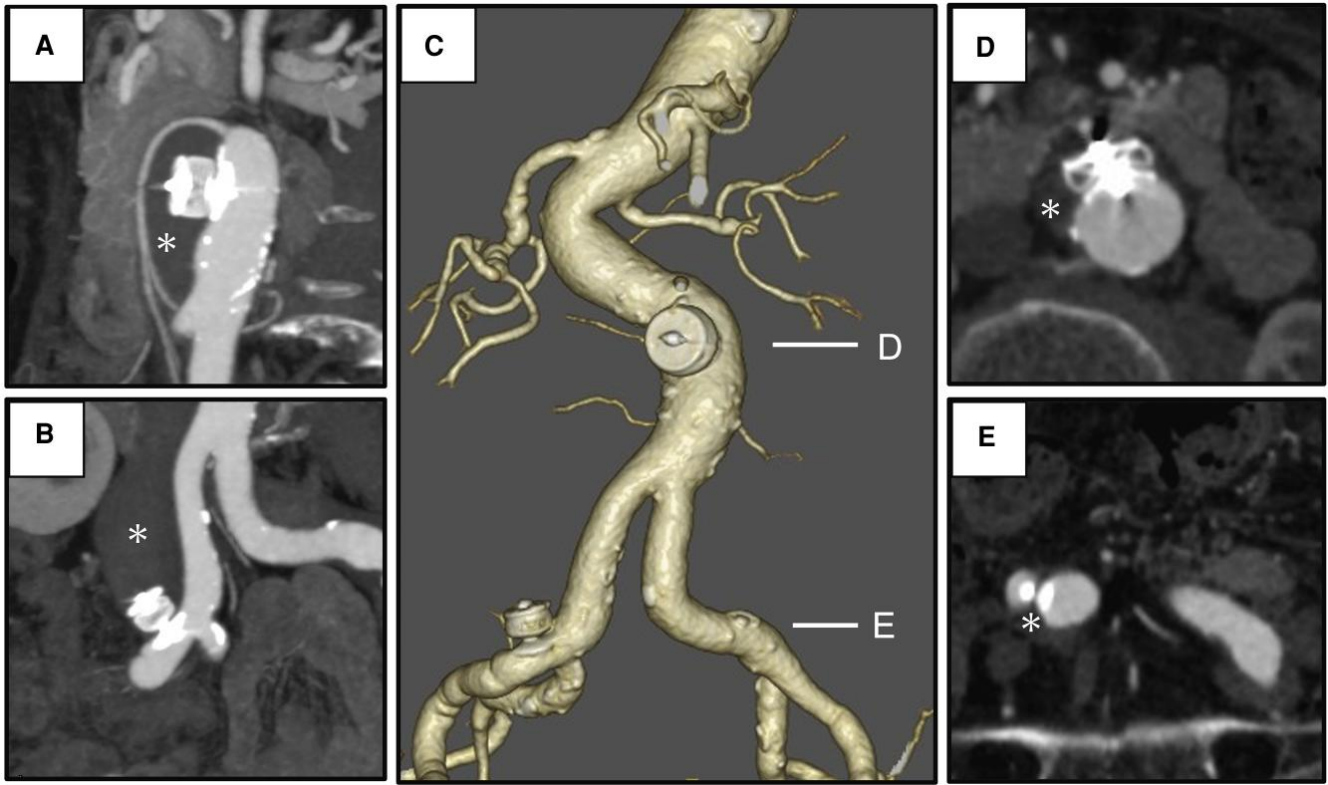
Follow-up contrast-enhanced computed tomography. A contrast-enhanced computed tomography performed 1 week after the procedure showed no blood flow into the false lumen (asterisk) of the abdominal aorta (*A*) and common iliac artery (*B*). Six months later, volume rendering confirmed no displacement of the two Amplatzer Vascular Plugs (*C*). The diameter of the infrarenal abdominal aorta reduced from 38 to 28 mm, while the right common iliac artery decreased from 34 to 22 mm. Additionally, the true lumen of the right common iliac artery expanded. Two years postoperatively, a computed tomography with contrast demonstrated significant regression of the false lumen (*D* and *E*). CIA, common iliac artery; CT, computed tomography.
